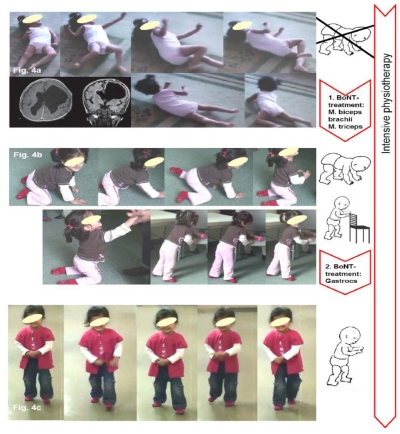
Case example of specific selection of the key-muscles: 3 year old girl with right-sided unilateral spastic cerebral palsy. (a) At first presentation, crawling as a motor milestone was impossible. The girl was able to roll from supine to prone position and *vice versa* with her right arm held in flexion due to spasticity. Hence, rolling was her form of moving about. No speech (not even single words) had developed. The neuropediatric diagnosis was spastic hemiplegia with global retardation. Despite a multi-level BoNT‑A injection, elsewhere performed, in the lower extremity, and physiotherapy on a regular basis, no improvement of motor development was achieved. Two sections of the cranial MRI are shown in the bottom left. The clinical examination revealed pronounced catch of the flexors, as well as the extensors of the right upper arm (Ashworth 3). Therefore, BoNT-A was injected in those muscles (30 units of Dysport^®^ each) and intensified physiotherapy according to the Berlin model was started. To reach the motor milestone, crawling extension and weight-bearing of both arms is necessary and a treatment goal. (b) 6 months after injection and intensive physiotherapy the motor milestone crawling was achieved. Standing and walking sideways holding on to objects is possible with a spastic equinus and a compensatory recurvation of the knee on the right side, as well as trunk inclination for balance and stability. To further improve the ability to stand and walk, the right M. biceps brachii and the right M. gastrocnemius were injected with 30 and 100 units, respectively, of Dysport^®^ and physiotherapy continued. A planned visit 4 months later is omitted. (c) At the next presentation one year later the patient is able to walk without support. She had attended physiotherapy on a regular basis. The girl is now able to speak simple sentences.
